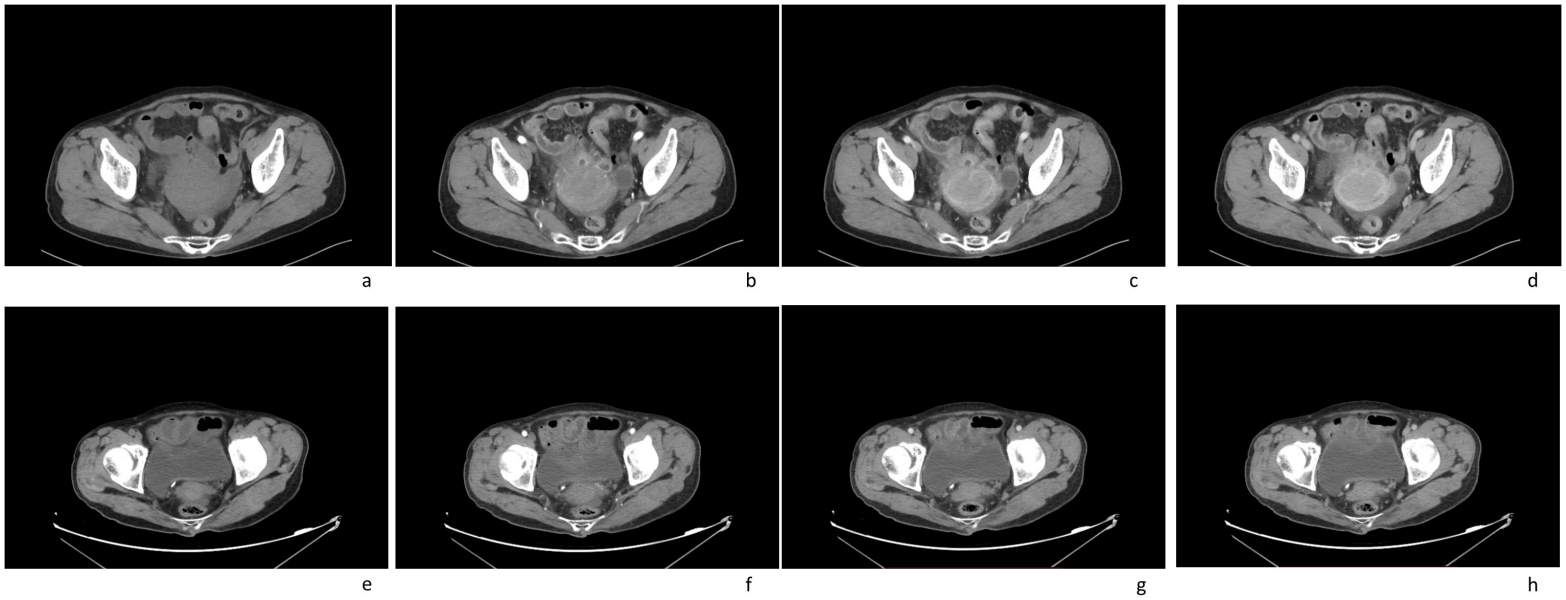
Computed tomography (CT) imaging scans of an 72-year-old female patient with a pelvic mass for more than 1 month: (A) plain CT imaging showed an enlarged uterine volume, soft tissue density shadow in the anterior wall of the uterus, with unclear boundary; (B–D) enhanced CT imaging(arterial phase[b]; venous phase[c]; delay phase[d]) showed that the lesion were slightly enhanced, the boundary was clear; (E–H) after treatment, enhanced CT imaging(arterial phase[f]; venous phase[g]; delay phase[h]) showed that the volume of the uterus had significantly reduced(plain CT imaging[e]).
